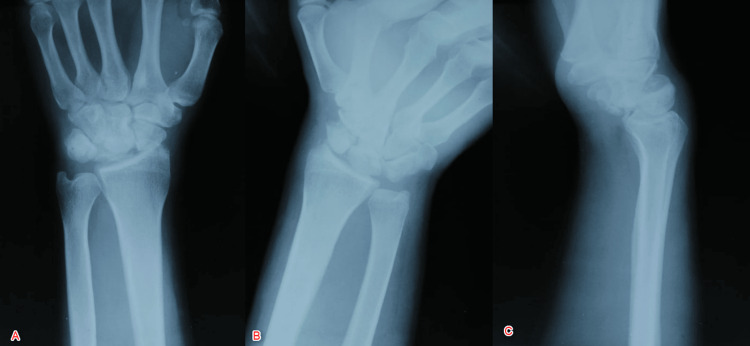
X-ray of the right wrist showing palmar dislocation of the capitate and distal scaphoid relative to the lunate A: Anteroposterior view; B: Oblique/Scaphoid view; C: Lateral view
